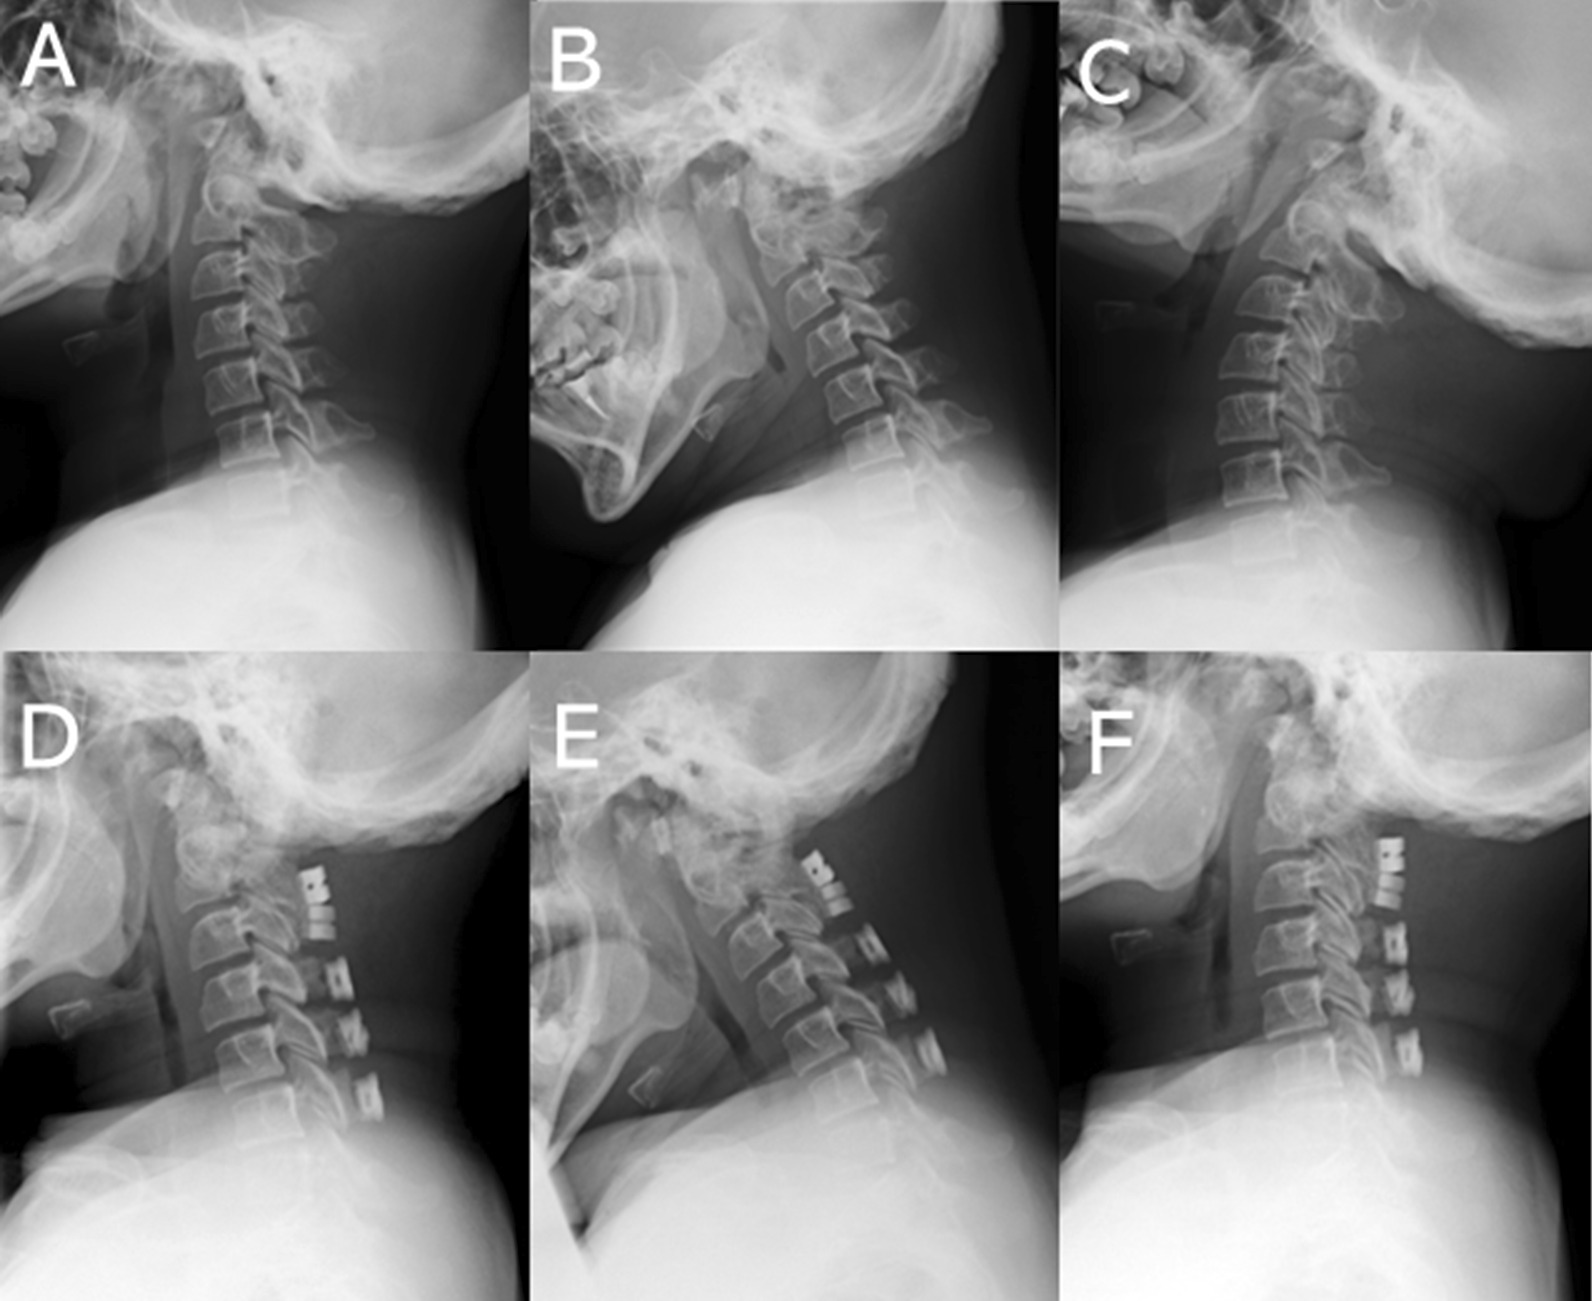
The cervical spine ROM after laminoplasty in a 19-year-old male patient with MPS type I. A–C Plain radiographs of the cervical spine before surgery at neutral, flexion (34˚), and extension (20˚) positions from the left. D–F Plain radiographs of the cervical spine at 4 years postoperatively at neutral, flexion (17˚), and extension (12˚) positions from the left. Approximately 50 % reduction of ROM was observed in this patient. * MPS* Mucopolysaccharidoses, *ROM* range of motion
